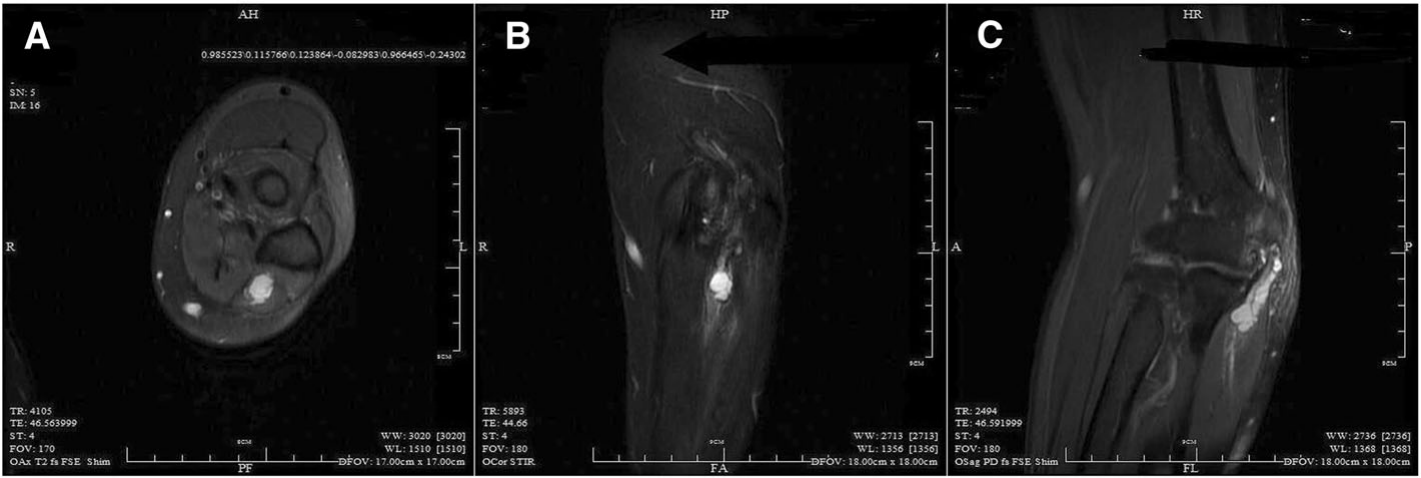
Display of magnetic resonance imaging before medical intervention. a Hyperintense lesion in ulnar nerve at cubital tunnel on T2-weighted image in transverse section. b Hyperintense lesion in ulnar nerve at cubital tunnel on T2-weighted image in coronal section. c Hyperintense beaded lesion in ulnar nerve at distal elbow on T2-weighted image in median sagittal section
